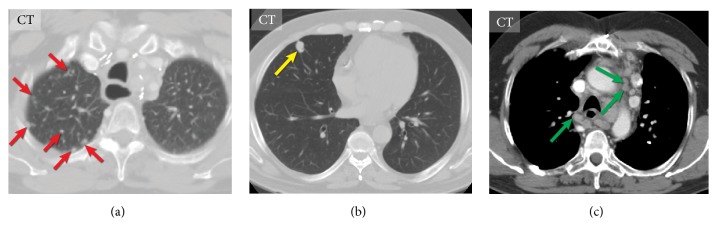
Computed tomography of the chest of different patients with metastatic medullary thyroid carcinoma. Disseminated pulmonary metastases (red arrows) (a). Solitary pulmonary metastases (yellow arrow) (b). Extensive metastatic mediastinal lymphadenopathy (green arrows) (c).
